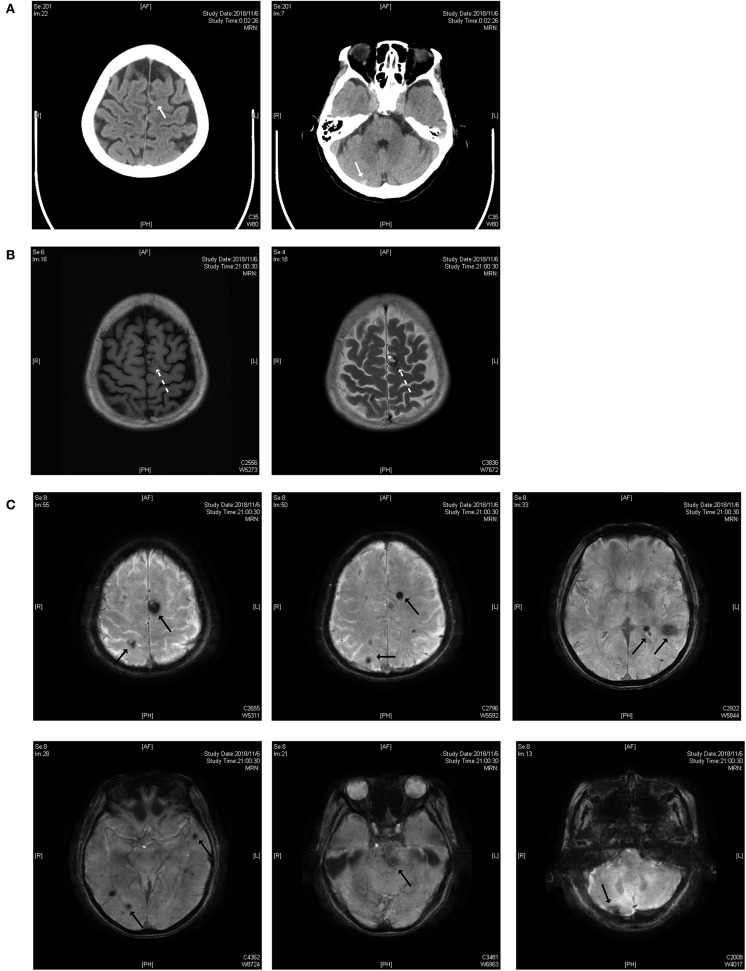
(A) Brain CT scan. The white solid arrows show a mixed-density lesion on the left parietal lobe and a high-density lesion on the right cerebellum. (B) T1 and T2 weighted images of the MRI scan. The dotted arrow indicates a popcorn-like mixed signal on T1WI and a high-signal lesion with a hemosiderin deposit surrounded on the T2 weighted image. (C) Susceptibility-weighted imaging (SWI). Black solid arrows indicate the lesions on the parietal lobe, temporal lobe, brainstem and cerebellum.
